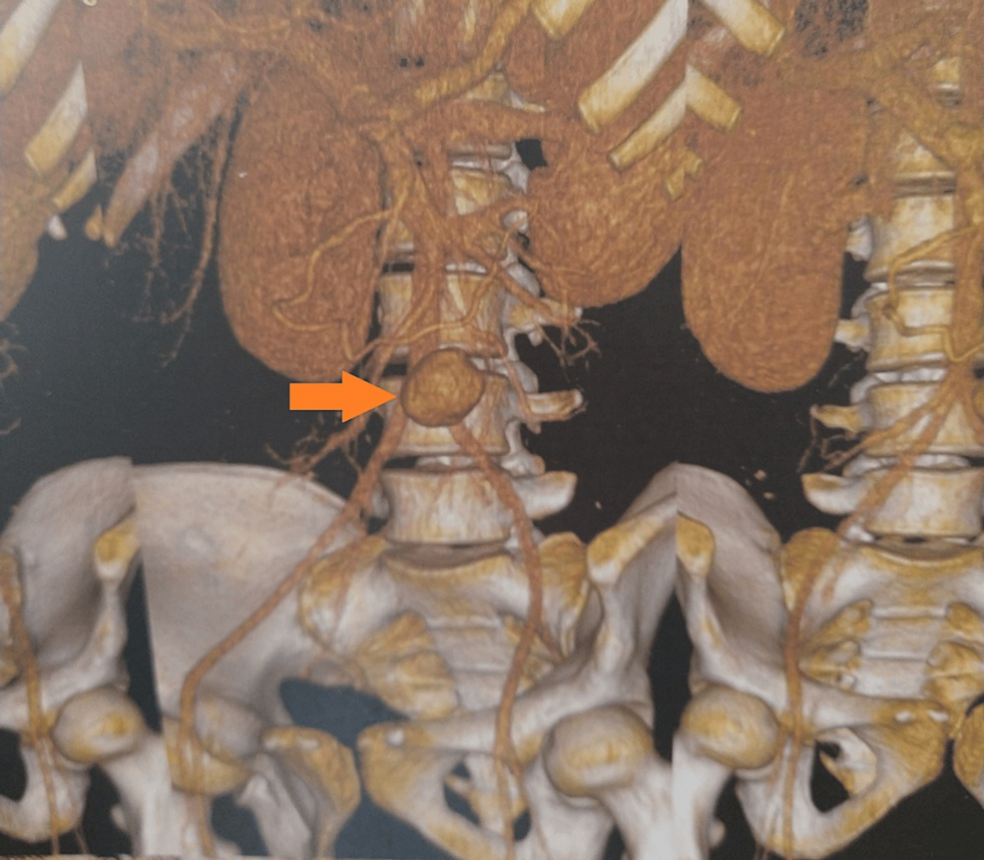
Abdominal CT angiography (3D reconstructive image) shows a pseudoaneurysm in the superior mesenteric artery located 9 cm distal to its origin (orange arrow).
